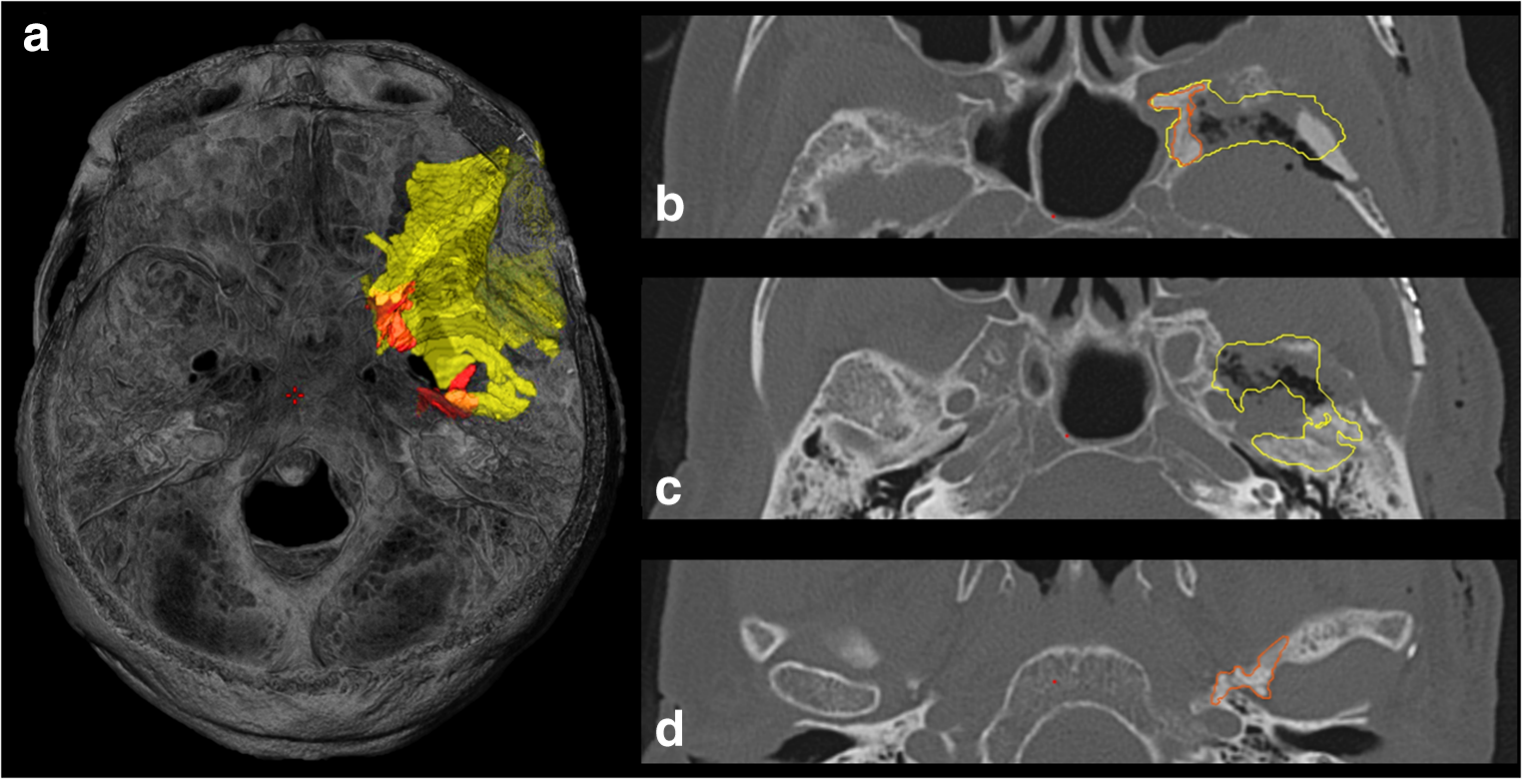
Operative result of case 54f by merging preoperative (*yellow*) and postoperative (*orange*) CT scans. a The osseous tumor remnants (*orange*) are mainly confined medial to the proposed line. b Removal of the lateral parts of the greater sphenoid wing. Resection was limited by involvement of the foramen rotundum and lateral sphenoid sinus wall. c Removal of the middle cranial fossa infiltrate lateral to the oval foramen. d Osseous remnant covering the TMJ pan medially and anteriorly
